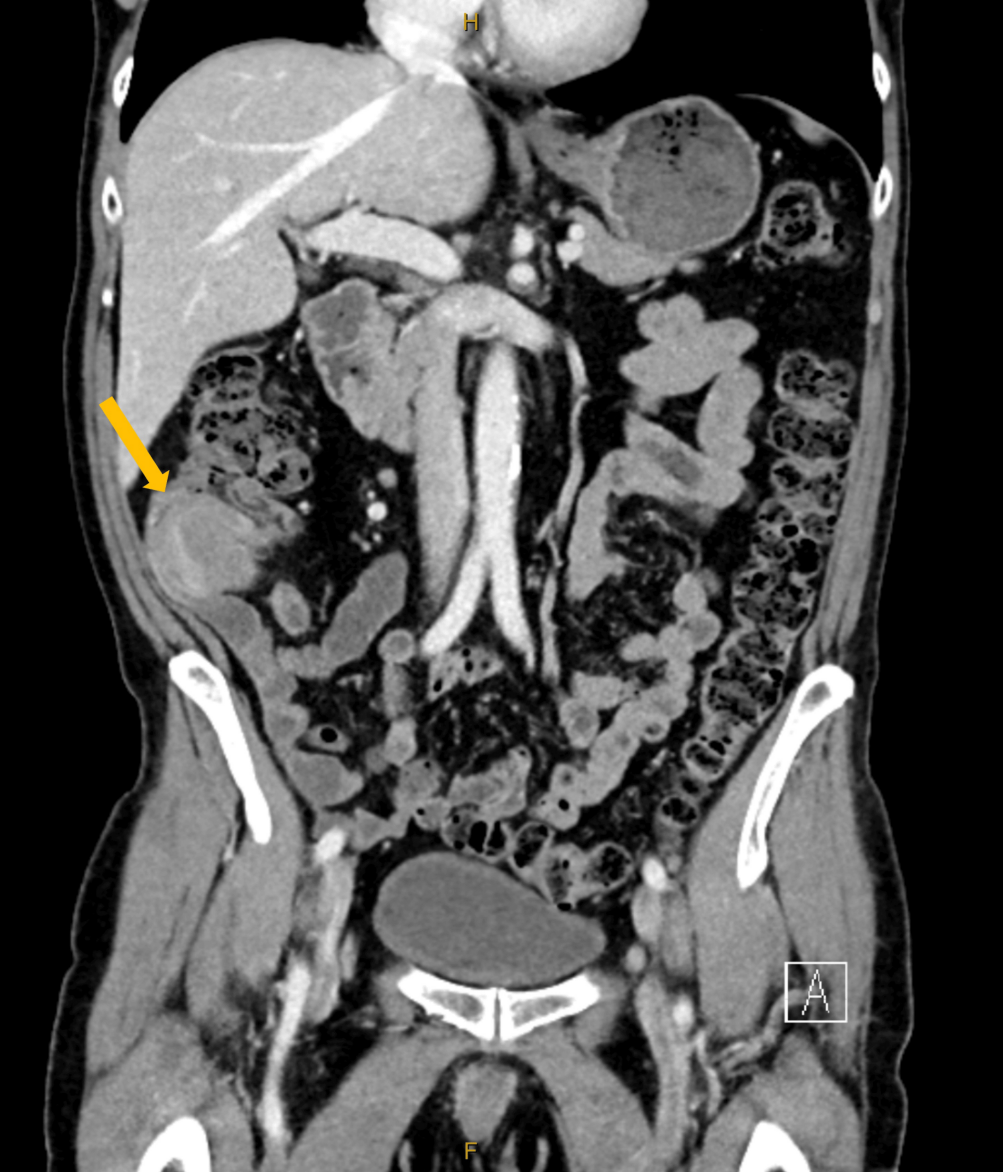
Initial computed tomography (CT) on presentation (coronal view) An arrow indicates intestinal intussusception in the right lower quadrant.
